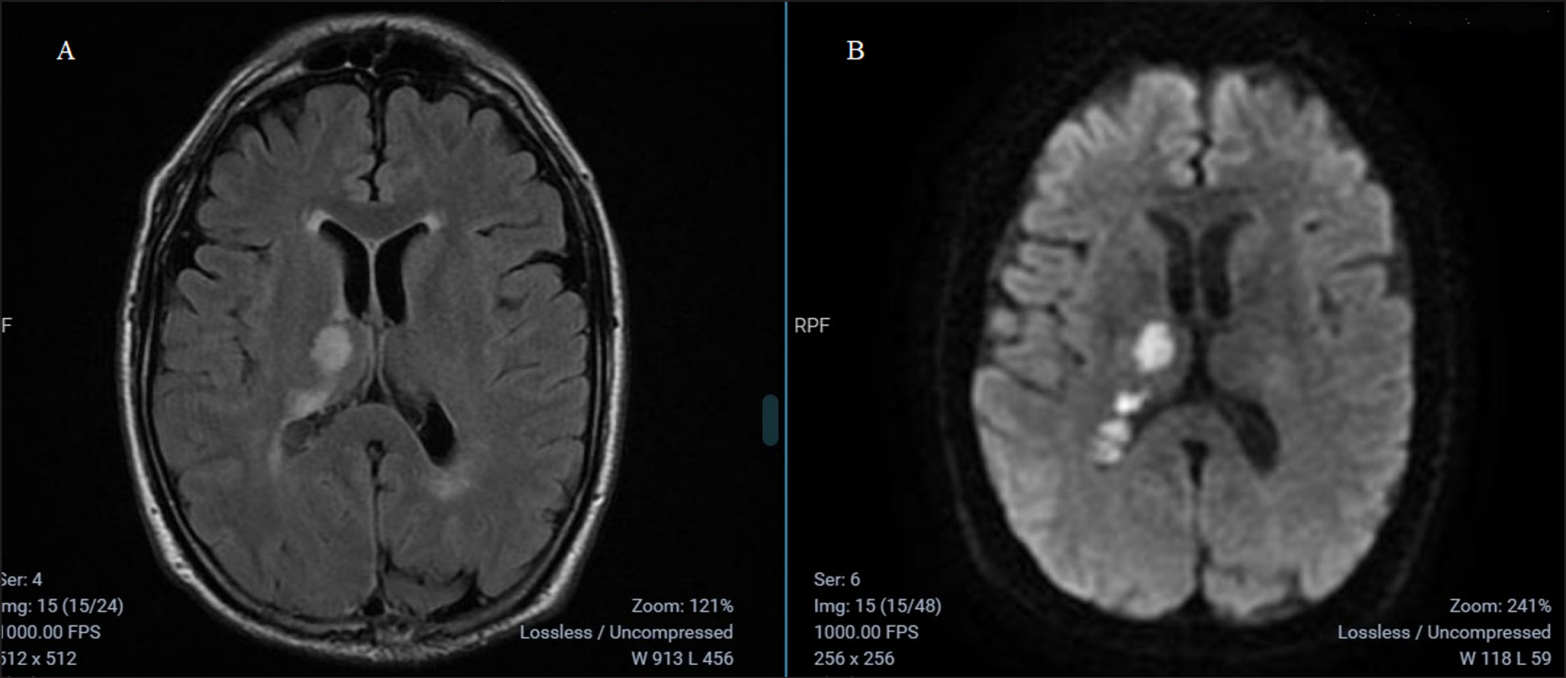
MRI images obtained on the 2^nd^ day of admission. MRI images showing signs of acute vascular insult on the right thalamus, marked by T2/FLAIR hyperintensities (A) and restricted water diffusion on DWI (B).
